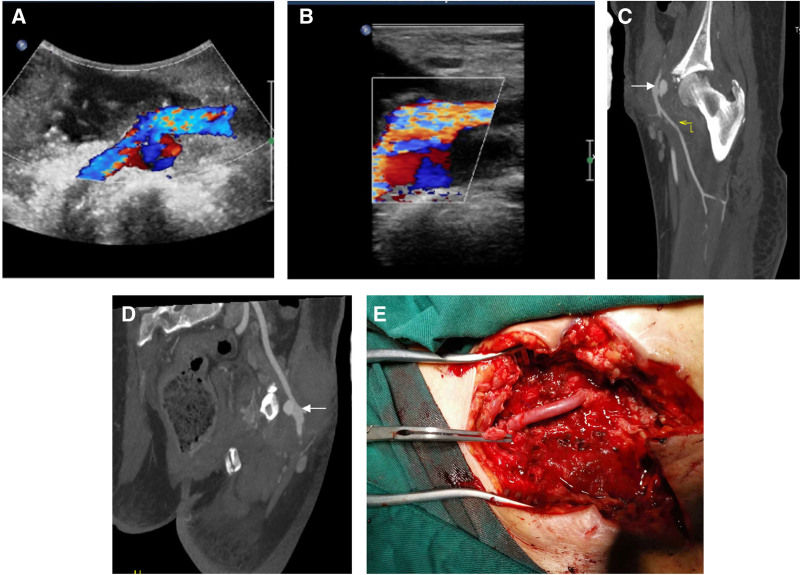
(A and B) The Doppler ultrasound image revealed a pseudoaneurysm formation in the left inguinal region with lumen narrowing and local intravascular thrombosis. (C and D) The computed tomography arteriography of the lower limbs revealed contrast leakage forming enhanced nodular shadows (white arrows), and active bleeding due to vascular rupture was considered. (E) After autologous saphenous vein grafting, there was no significant bleeding from the anastomosis and the artery pulsed well.
